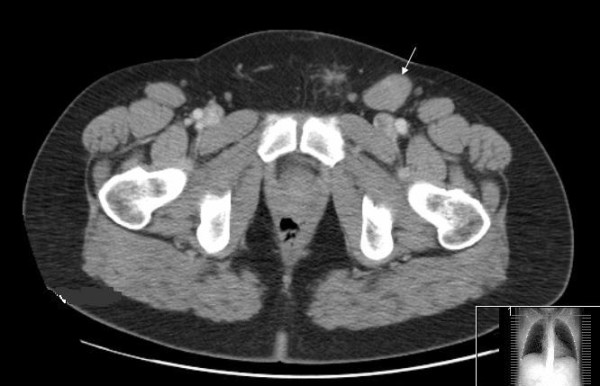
A CT scan of the pelvis revealing a 2.4 cm left inguinal lymph node (arrow).
